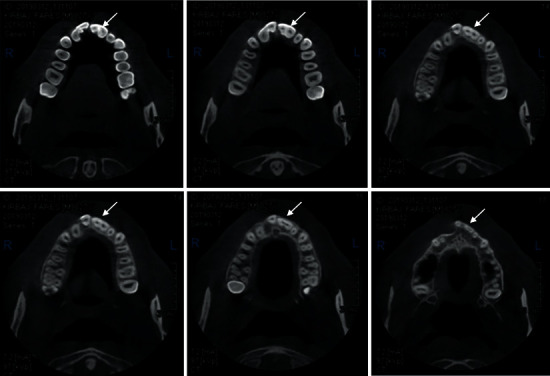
Plane of axial CBCT sections of maxillary showing fusion between #11 and #12 and a gemination in #21. The gemination presents a separated pulp chambers and merging root canals (arrow).
